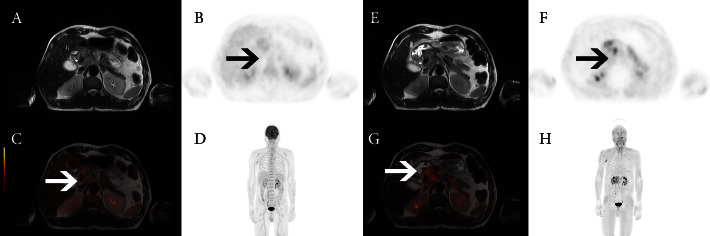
A 63-year-old male patient with gastric cancer, a gastroscopy showed that gastric cancer had invaded the lower esophagus, with perigastric infiltration. PET/MRI showed abnormal increases (arrow) in FDG metabolism (A-D) and FAPI uptake (E-H).
